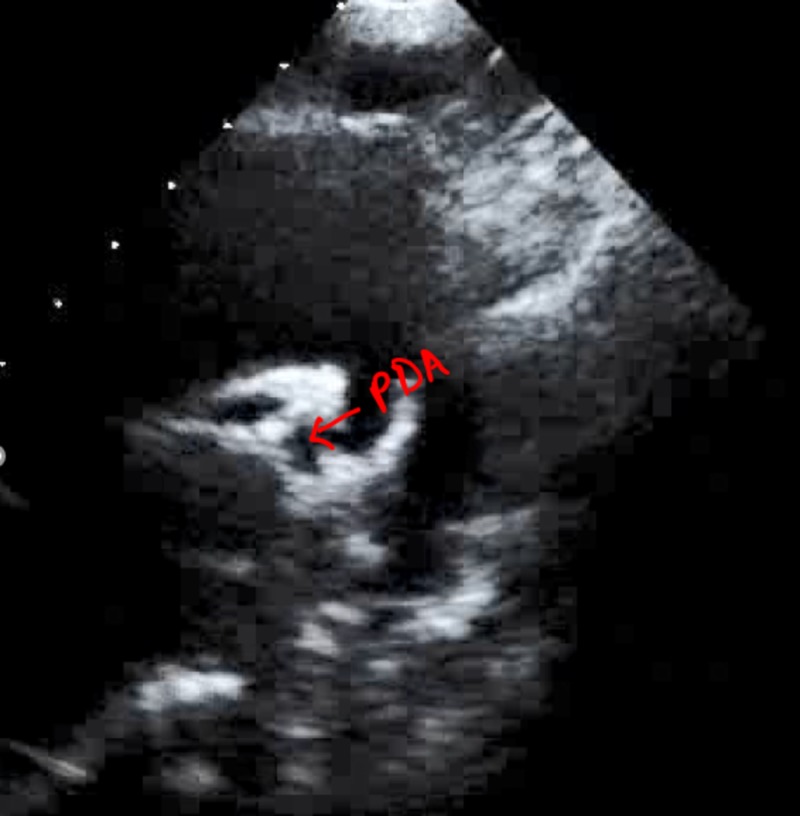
ECHO showing PDA ECHO, echocardiography; PDA, patent ductus arteriosus
